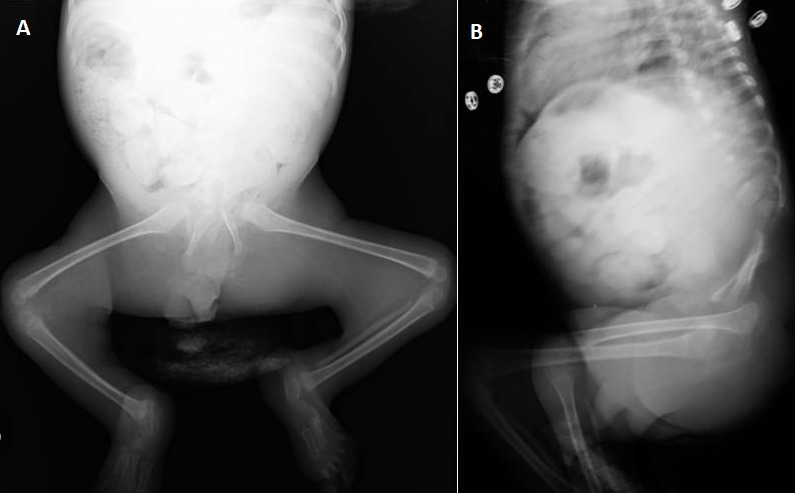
(A) radiographie standard de face du bassin et des membres inférieurs montrant un bassin rétréci en entonnoir avec raccourcissement des fémurs, abduction des cuisses et flexion des genoux réalisant la position en « frog-like » des membres inférieurs; (B) radiographie du rachis dorso-lombo-sacré de profil met en évidence une agénésie sacro-coccygienne et de la dernière vertèbre lombaire
